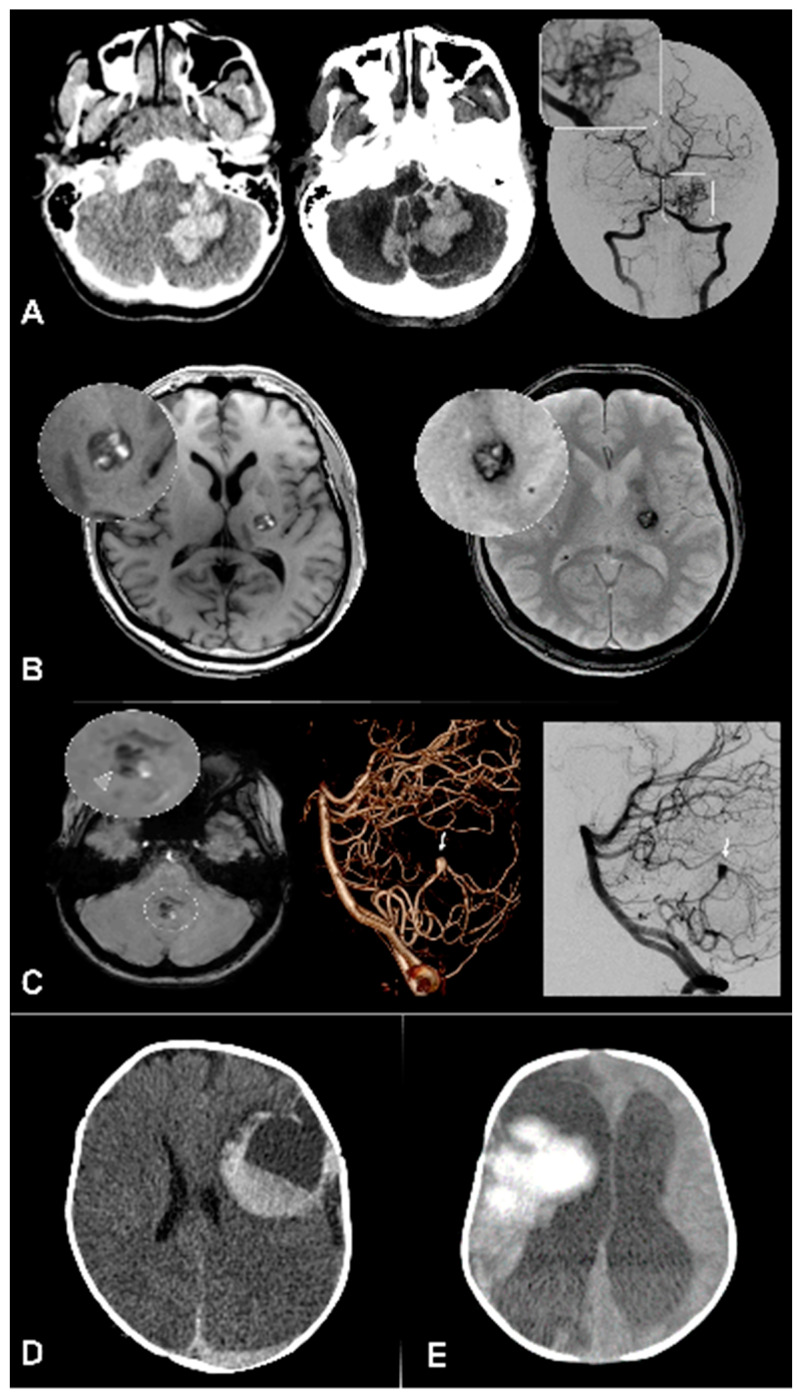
Pediatric intracerebral hemorrhage (ICH) main etiologies. (A) Left cerebellar mid peduncular hemorrhage, demonstrated on computed tomography (CT) and CT-angiography; digital subtraction angiography (DSA) (right and inset) shows malformative vessels with PICA and AICA feeders, a nidus and deep venous outflow corresponding to an arteriovenous malformation. (B) Left internal capsule cavernoma, with distinctive heterogeneous signal on T1 (left) and hyposignal on T2 * (right). (C) Smaller ICH in the cerebellar vermis (arrowhead). DSA shows a fusiform, likely dissecting, aneurysm. (D) Clotting factor deficiency revealed by a left supratentorial bleed. The presence of a fluid level is suggestive of a coagulation deficit. (E) Hemorrhagic transformation of a large right hemispheric ischemic stroke.
